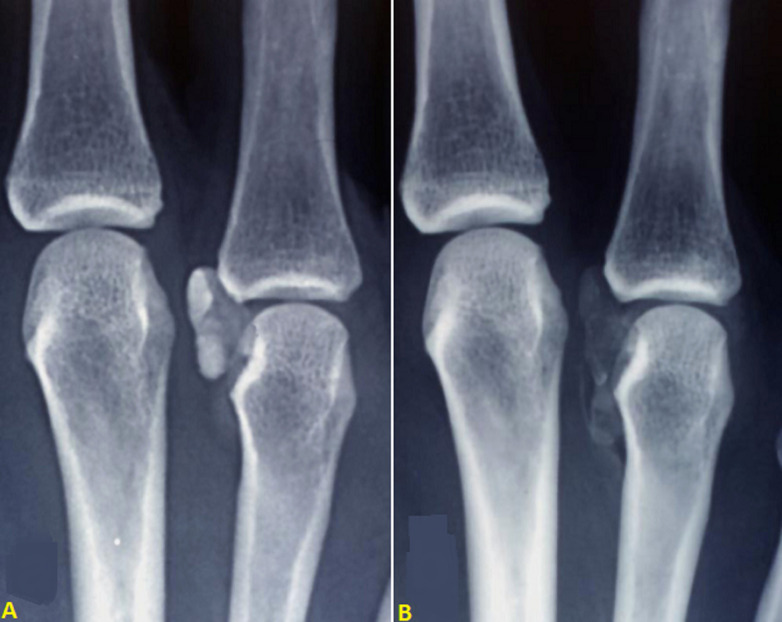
anteroposterior radiograph centered on the inflammatory joint; A) radiographic appearance in the acute phase (beginning of resorption); note the clear outline and periarticular location of the calcification; B) appearance 3 weeks later; gradual disappearance with spread of the calcification and attenuation of its calcific density
